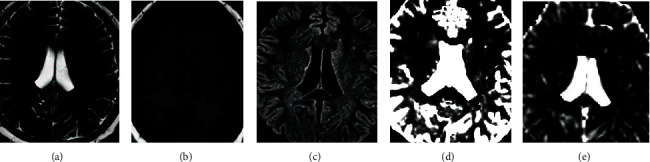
Imaging data of a patient with HCI. (a–e): T1WI, T2WI, pressurized-water T2WI, DWI, and FLAIR, respectively.
